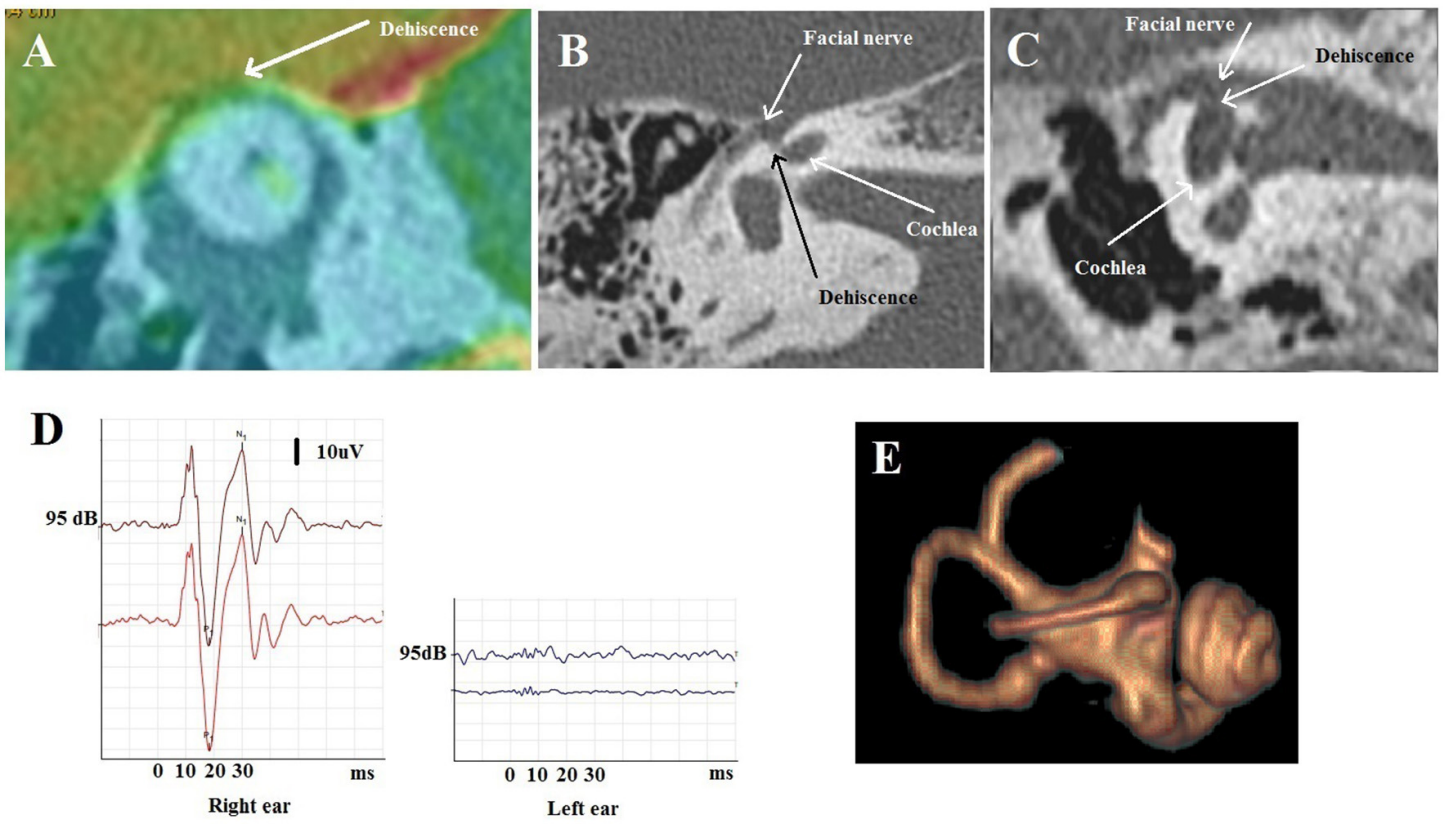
Multiple localization OCD (case report 2). (A) Fusion between HRCT and 3D T1 weighted contrast enhanced sequence in Poschl plane: right SSCD. (B,C) Temporal HRCT in axial (B) and coronal (C) plane: right CFD. (D) oVEMPs before surgery with large amplitude on the right side. (E) After surgery: labyrinthine MRI: partial amputation of the anterior arm and the top of the right SSC.
